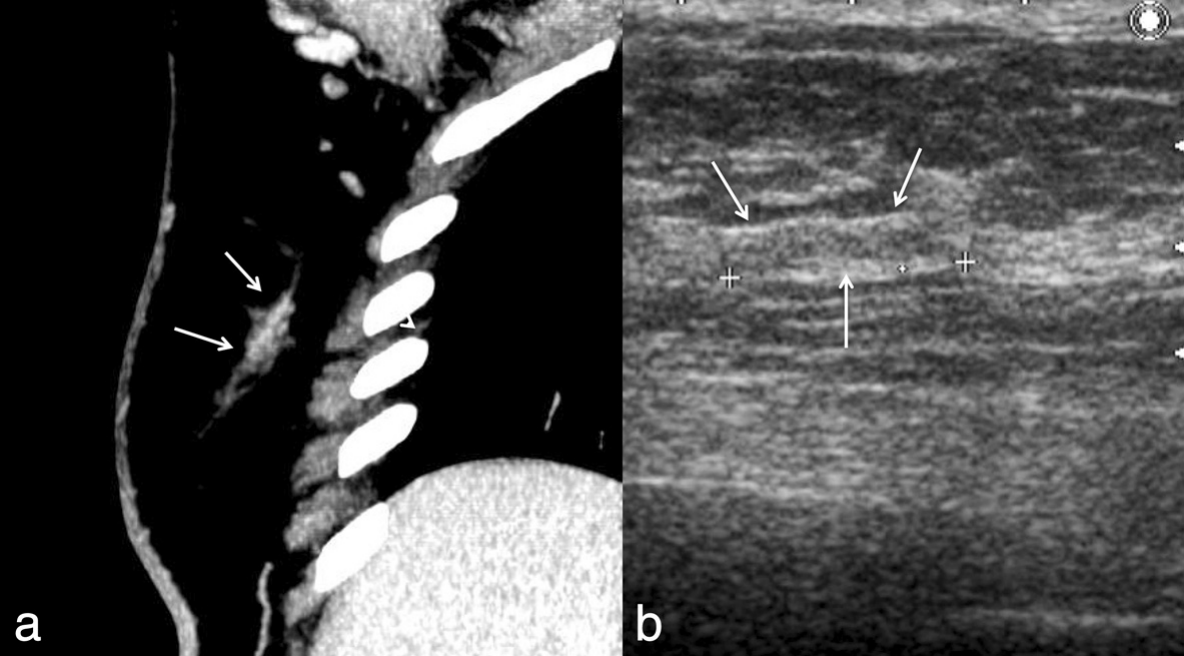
MDCT showed segmental enhancement on the ipsilateral breast. An enhanced lesion was depicted in the thin breast gland. Targeted sonography detected a hypoechoic lesion in the thin breast gland, the size of which was 14x2 mm. Excisional biopsy was performed for definitive diagnosis. Pathological diagnosis was ductal carcinoma in situ.
